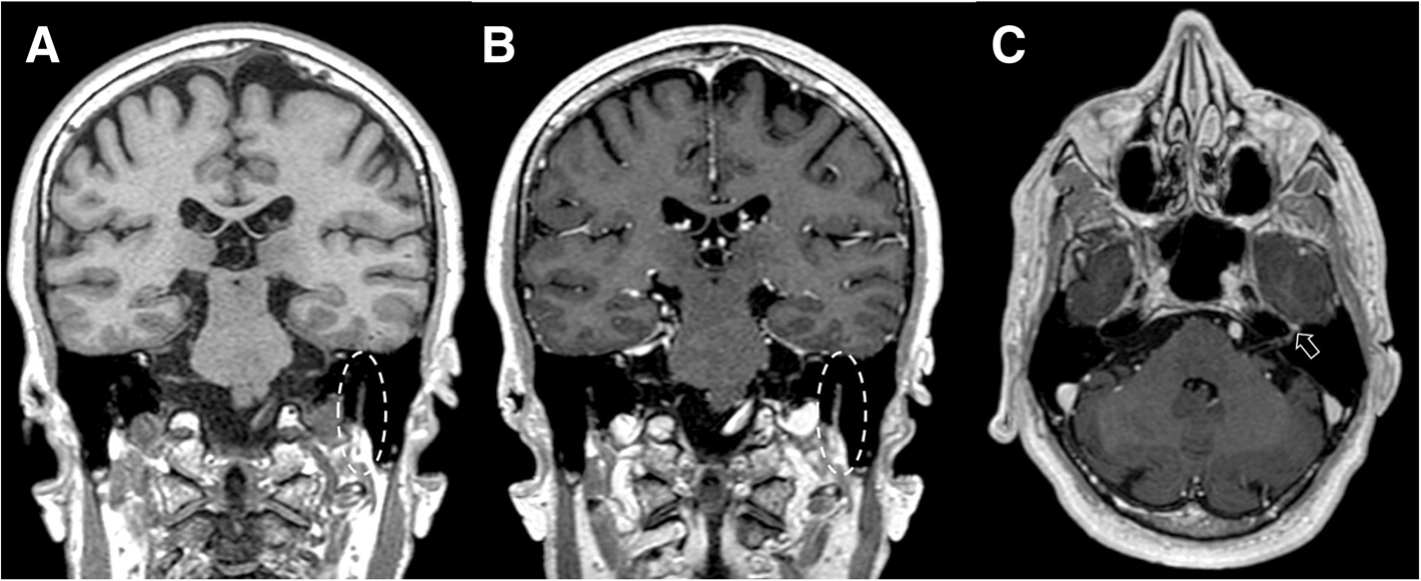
Bell’s Palsy. MRI T1-weighted pre- (a) and post-gadolinium (b), coronal images; T1-weighted post-contrast axial image (c). In a, b, note the swelling and enhancement of the left facial nerve (dotted circles); in c, the enhancement around the geniculate ganglion (arrow)
